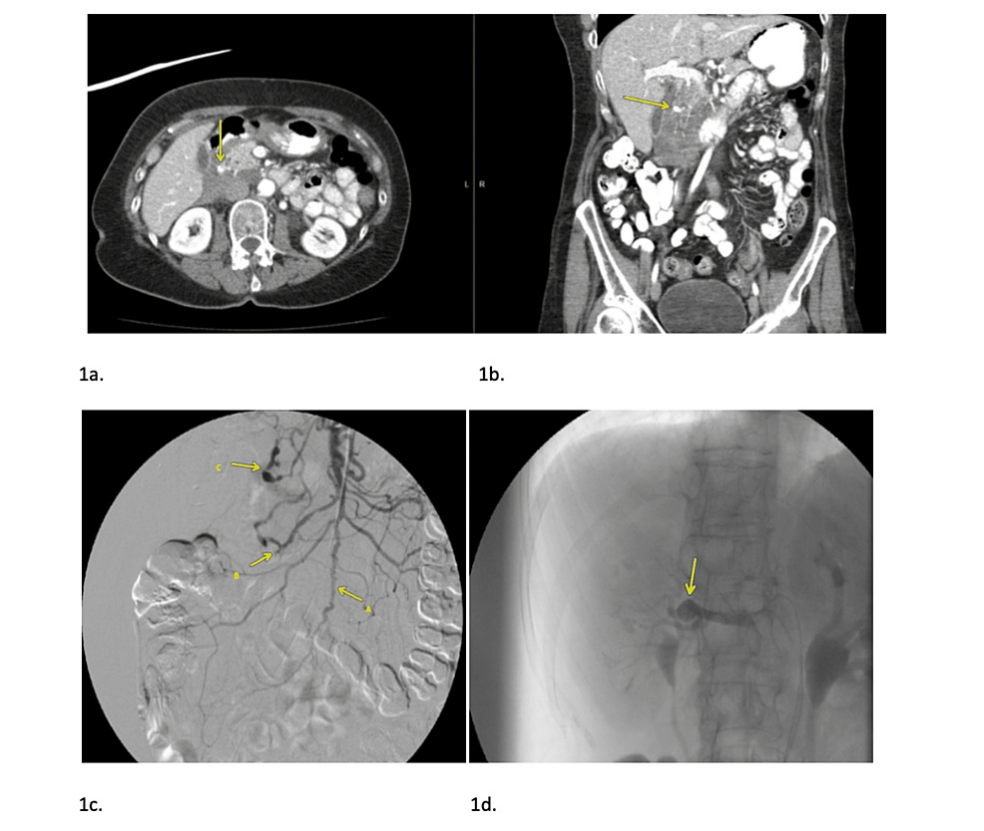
1a - Axial and coronal reformatted images from a contrast enhanced CT examination day 1 of admission demonstrating peripancreatic hemorrhage (arrow). 1b - Rounded foci of high density material (arrow) highly suspicious for peripancreatic pseudoaneurysm. 1c - Superior mesenteric artery (SMA) arteriogram from day 1 demonstrating diffuse irregularity of a jejunal branch of the SMA (Arrow A) as well as multiple stenoses and luminal irregularity of SMA branches (arrows B and C). 1d - Arteriogram of the right renal artery demonstrating a 2.7 cm aneurysm of the renal artery at the renal hilum (arrow).
